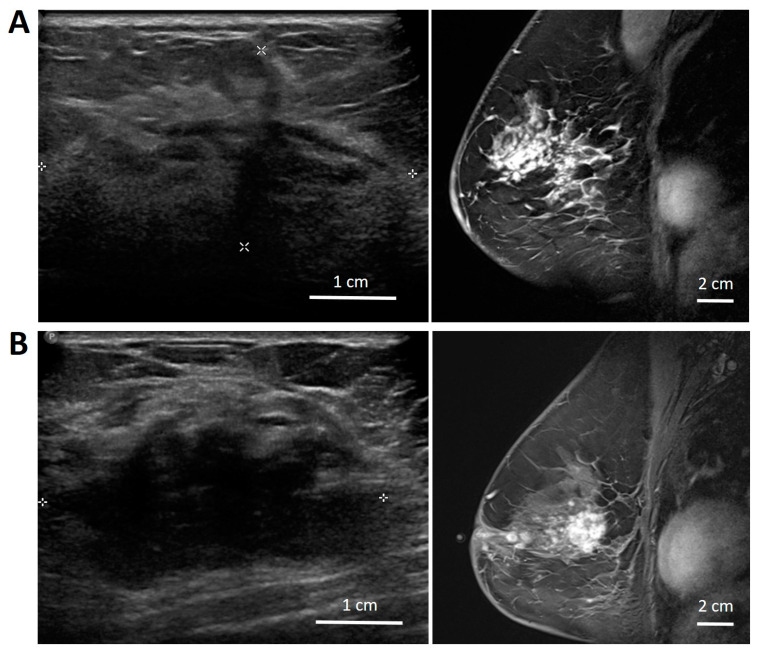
Example of an ultrasound (left) and MRI (right) images of patient 12 (A) and patient 19 (B) showing a tumour with heterogeneous, distorted, and poorly defined boundary structures.
